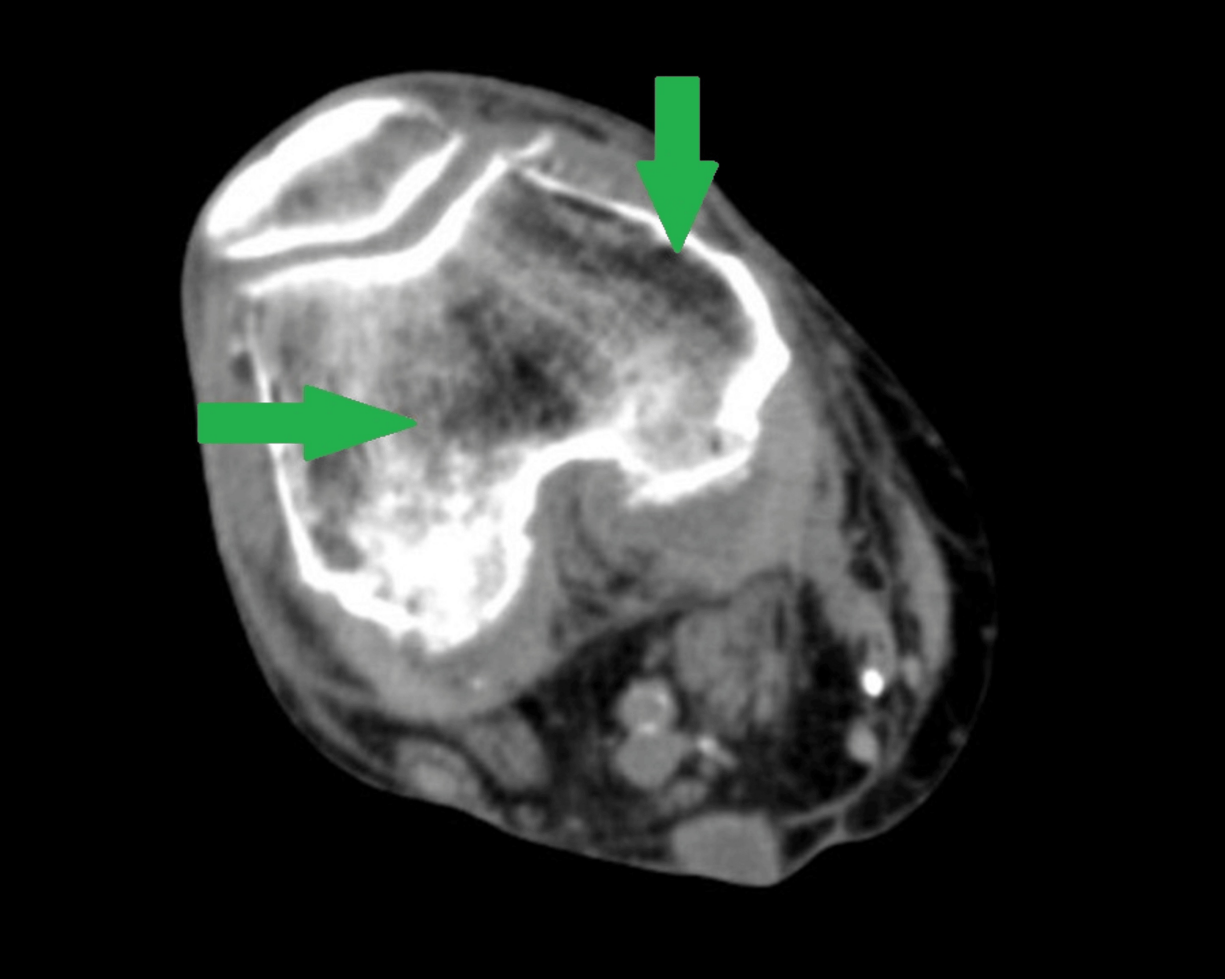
CT of right lower extremity showing joint effusion (green arrows) of the right knee
